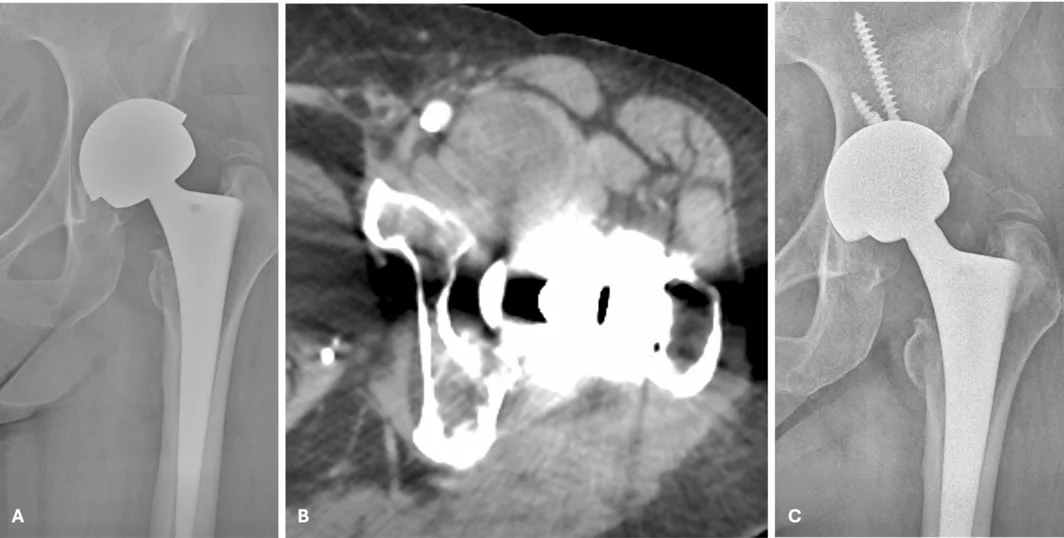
Imaging studies and surgical steps from a patient with a pseudotumor of the hip treated using a one‐stage approach. (A) Preoperative plain antero‐posterior X‐ray and (B) axial CT scan with contrast of a 58‐year‐old female patient treated 7 years earlier with a MoM hip prosthesis for primary hip arthrosis. Pre‐operative CRP was within normal range, and the stool testing was negative for bacteria. The patient was considered for a one‐stage approach. (C) Post‐operative plain antero‐posterior X‐ray taken 12 months after the acetabular revision.
